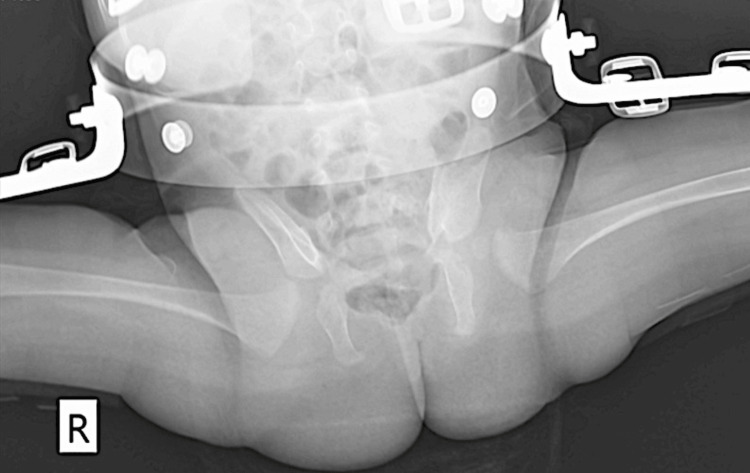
Pelvic radiograph demonstrating hip stabilization using a Hilgenreiner abduction brace
